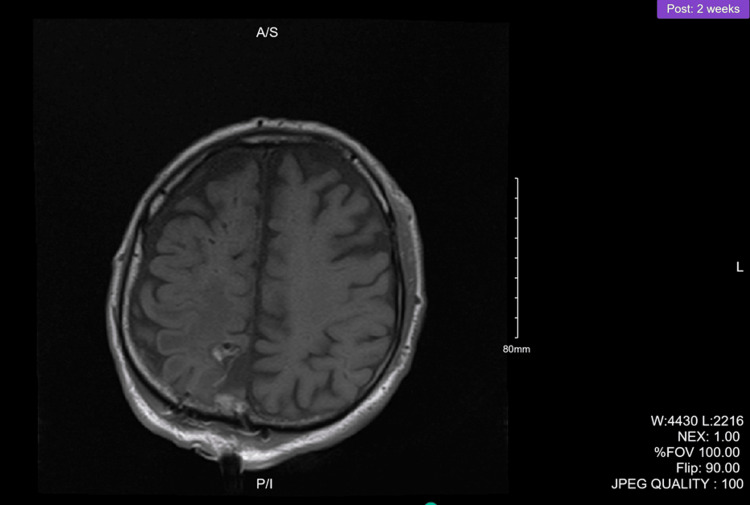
Postoperative brain MRI coronal view MRI: magnetic resonance imaging
